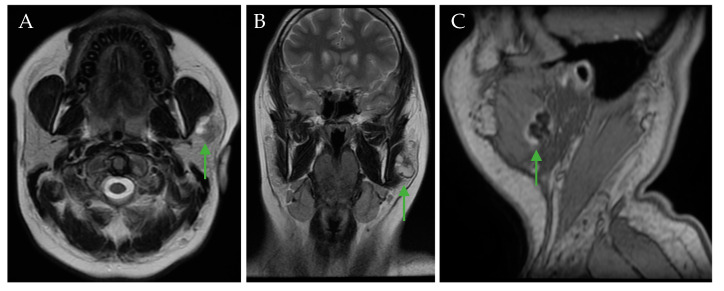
MRI sections showing an apparently well-circumscribed left parotid gland mass (green arrows): axial T2 FSE (fast spin-echo sequence) (A), coronal T2 FSE (B), and sagittal MPR T1 (Multiplanar reconstruction) (C).
